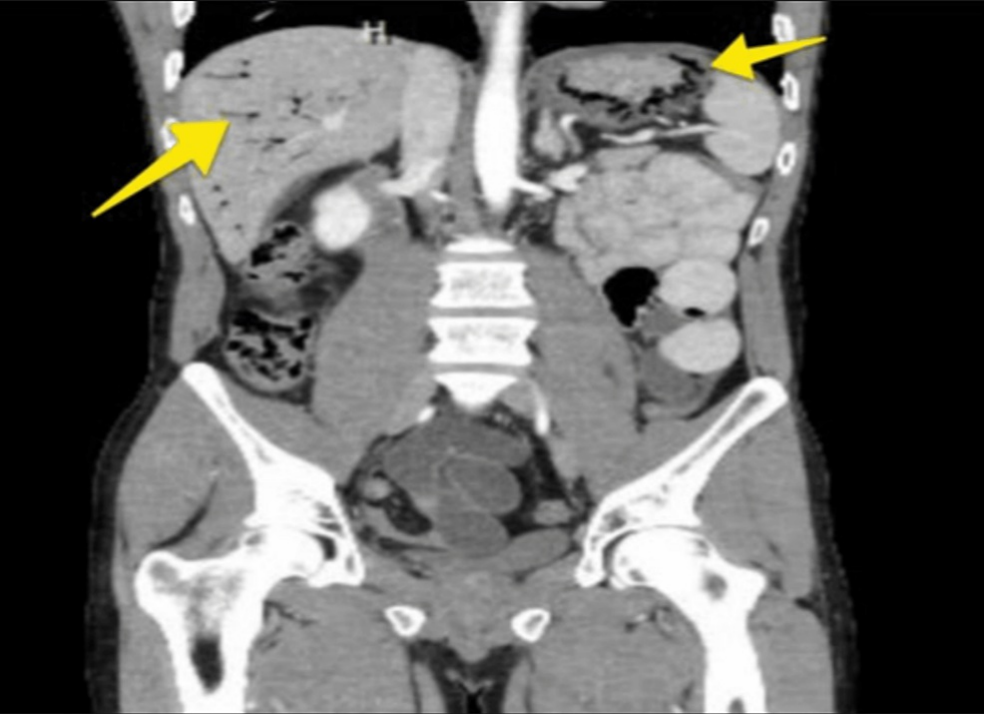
Coronal view of CT scan of the abdomen and pelvis with contrast showing gastric pneumatosis (right arrow), proximal duodenum pneumatosis, and portal venous gas (left arrow)
